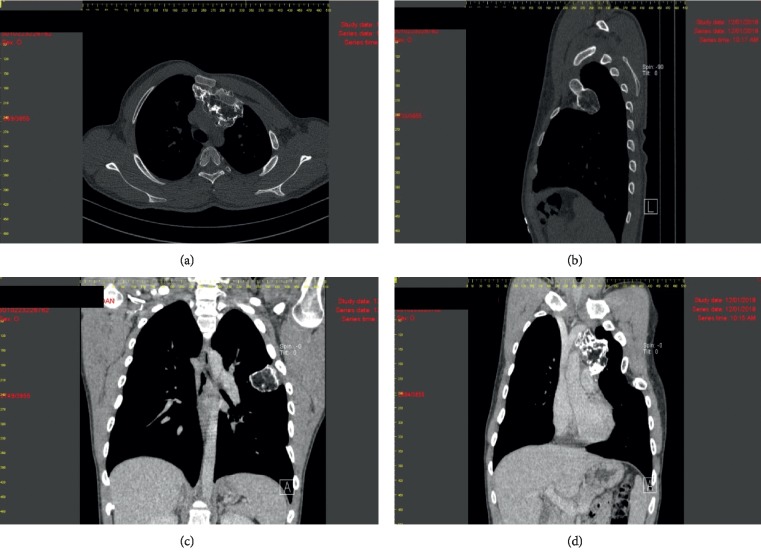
Thoracic computed tomography (CT) scan. (a) Osteochondroma developed on the internal face of left ribs, anterior arch, with mass effect on anterior mediastinum, which is associated with rib cage deformation (axial section, bone window). (b) Osteochondroma developed on the internal face of left ribs, anterior arch (sagittal section, bone window). (c) Osteochondroma developed on the internal face of left ribs, lateral arch (coronal section, bone window). (d) Osteochondroma developed on the anterior arch of left ribs, with preaortic expansion in the retrosternal space and ascending aorta compression.
